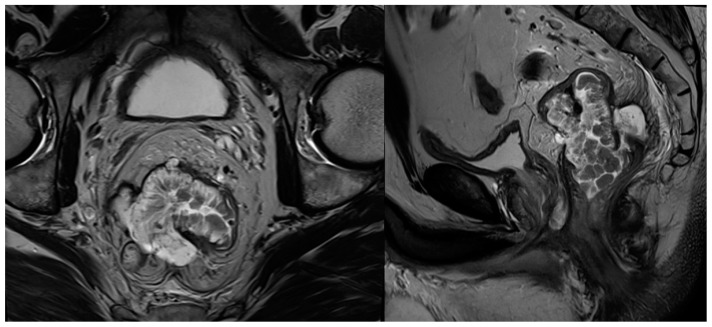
T2-weighted MRI in axial and sagittal planes of a rectal tumour demonstrating extensive high-T2 signal mucinous component.
